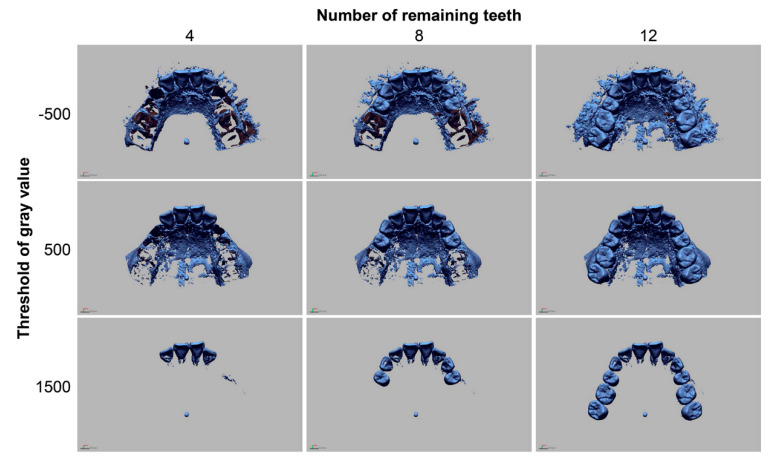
3D reconstruction images of cone-beam computed tomography in the different conditions of the threshold of gray value of segmentation and remaining teeth.
